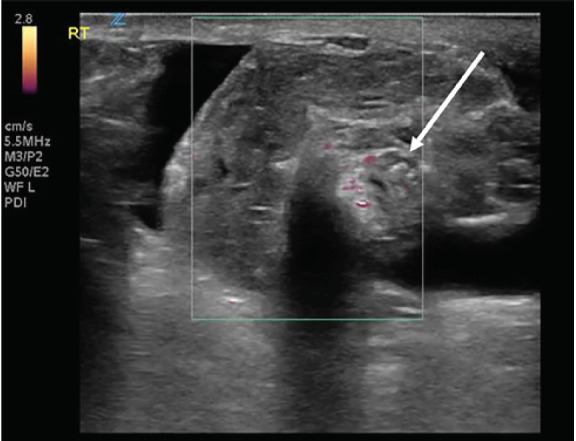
Point-of-care ultrasound of the spermatic cord demonstrating “whirlpool sign” (arrow) and diminished vascular flow on power Doppler ultrasound.
